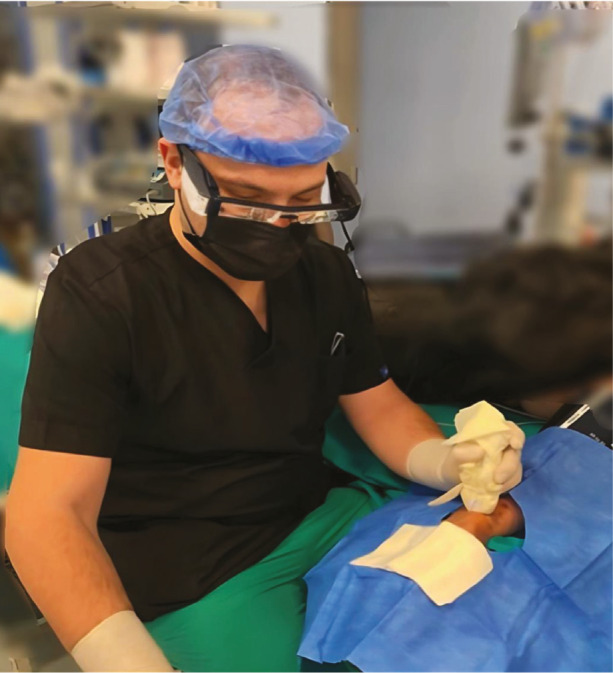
Radial artery catheterization with smart glasses integrated USG. USG, ultrasonography.
